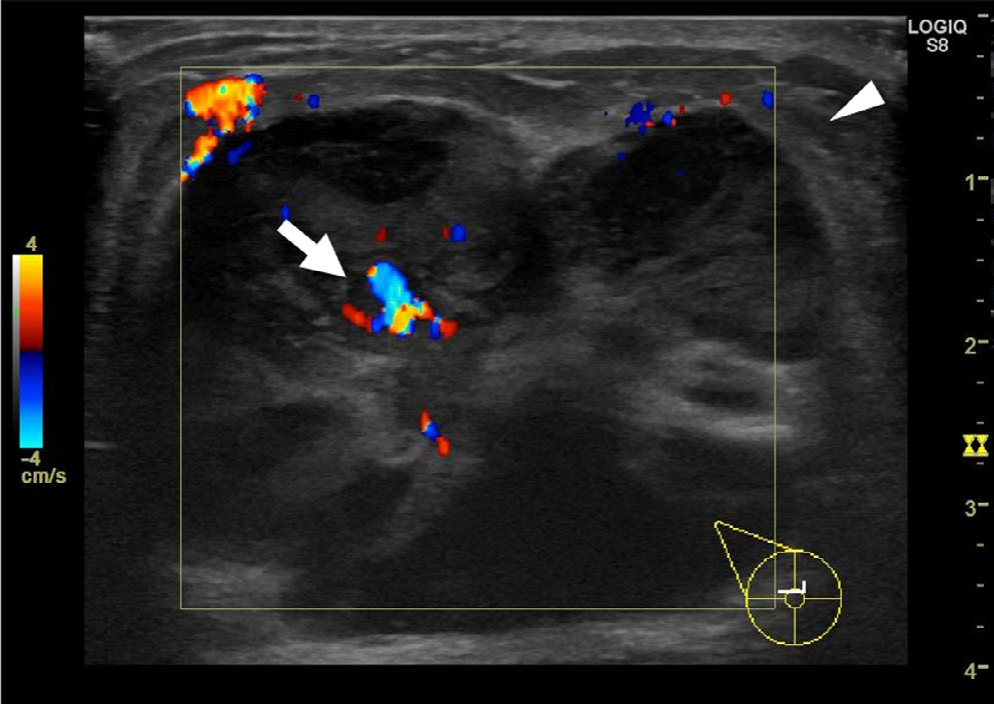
Ultrasonography. A 47‐mm irregular hypoechoic mass with heterogeneous internal echo (arrowhead) and blood flow in the mass (arrow)
